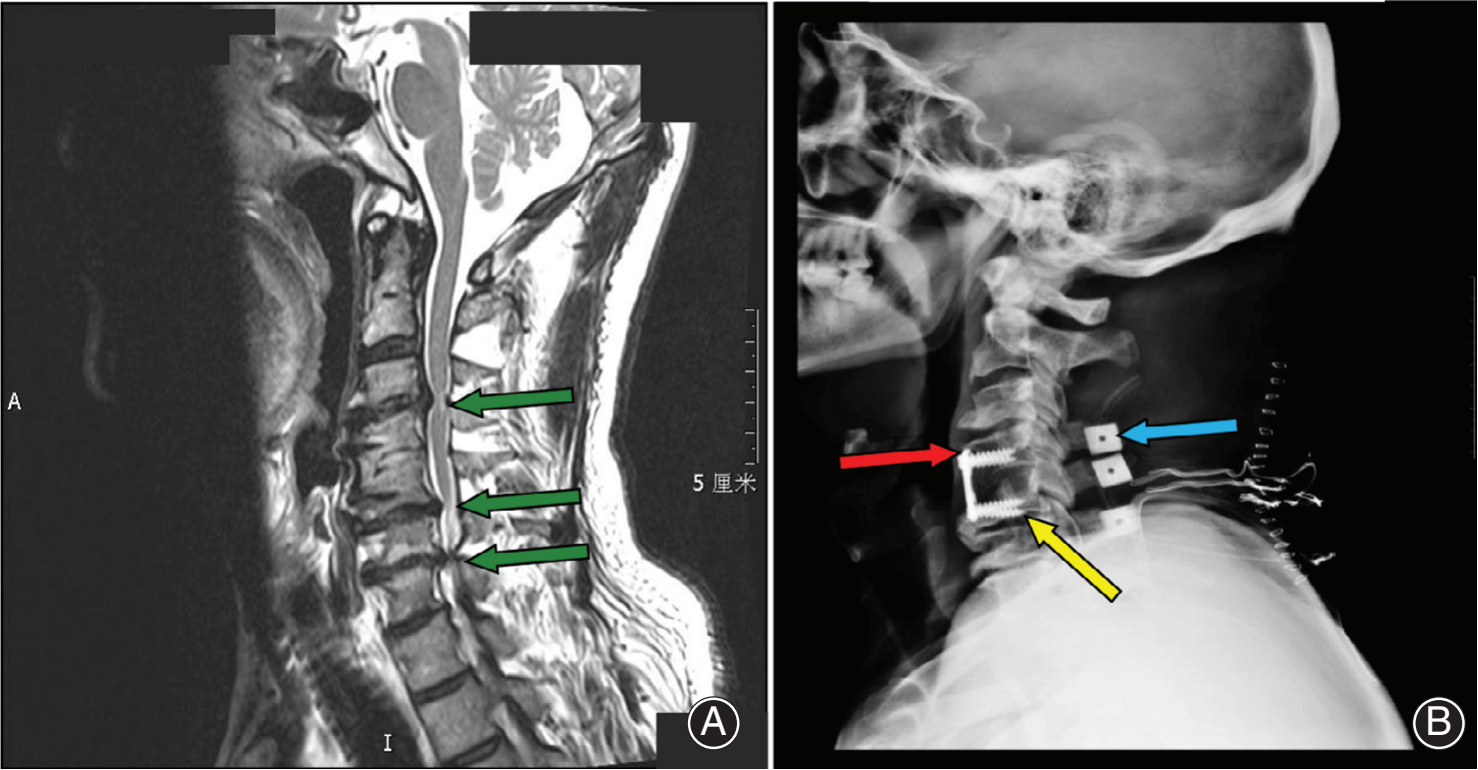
Preoperative and postoperative radiological imaging scans of a patient. (A) The first postoperative MRI imagine. Cervical MR scan obtained after the first C_4/5_ ACDF. Postoperative scan showing ASDis at C_3/4_ and C_5/6_‐disc herniation, corresponding to the flat spinal cord compression (green arrow). (B) The second postoperative X‐Ray imagine. Radiograph obtained after the reoperation (SLAC). The distance between the vertebral plate and the lower edge of the C_3/4_ intervertebral space is <5 mm (red arrow). The C_5_ vertebral body screw has broken through the C_5_ vertebral endplate and the C_5/6_ annulus (yellow arrow). The figure shows that there is an artificial bone (blue arrow) between the C_4_, C_5_, and C_6_ spinous processes.
